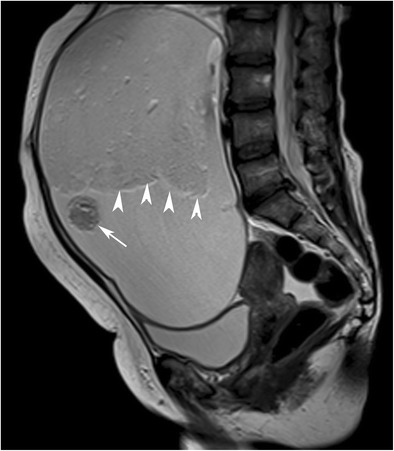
Sagittal T2-weighted MR image of a 56-year-old female (same case as in Fig. 15) with mature cystic teratoma shows chemical shift artefact (arrowheads) in the interface of two different hyperintense layers. Note that the complete gravity-dependent layering of the fatty component in the cranial part is not formed regarding the high viscosity. A floating rounded structure is also seen in the cystic lumen (arrow)
